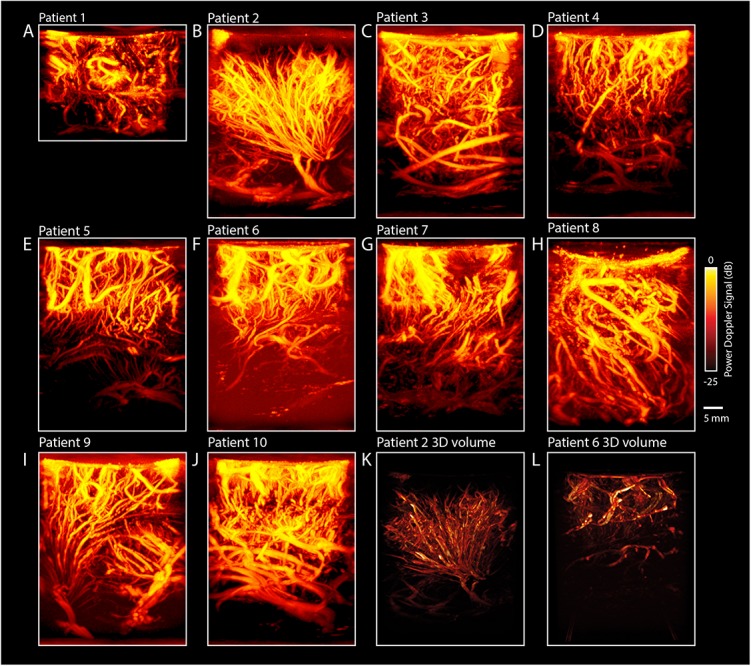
Maximum Projections of all patients (*n* = 10). (A–J) In addition to the regular 2D-images, we also made maximum projection images, showing an overview of the maximum signal per pixel during the imaging session of 60 s. As such, a single image with more depth-information can be created. For each patient, the most vascular-dense pre-resection image is highlighted, revealing a rich diversity in vascular patterns across patients. (K) 3D-overview of the pre-resection tumor vasculature images as acquired intra-operatively for pt.#2. Pre-operative MRI showed a suspected LGG tumor in the left frontal lobe. The probe was moved over the tumor along a continuous trajectory. During linear 2D-acquisition, an arborization structure was observed of the vessels within the tumor. The arborous vascular structure seems to originate from a single point, dubbed the *vessel of origin*. Multiple 2D PDIs acquired during the 60 s measurement session, showed the arborous structure. The PDIs were stacked offline in a 3D-volume, which confirms the vessel of origin, as depicted here. See also Supplementary Video S4 for the 3D reconstruction. (L) Overview of the pre-resection tumor vasculature images as acquired intra-operatively for pt.#6. Pre-operative MRI showed a suspected LGG in the left occipito-parietal region. The probe was moved over the tumor along a continuous trajectory. During linear 2D-acquisition, a well-defined vascular structure was observed, delineating the tumor from the rest of the tissue. Multiple 2D PDIs acquired during the 60 s measurement session, were stacked offline in a 3D-volume, confirming the well-defined vascular area. See also Supplementary Video S5 for the 3D reconstruction. These last two examples highlight the potential of vascular mapping for brain-tumor delineations. fUS, functional ultrasound; LGG, low grade glioma; PDI, power doppler image.
